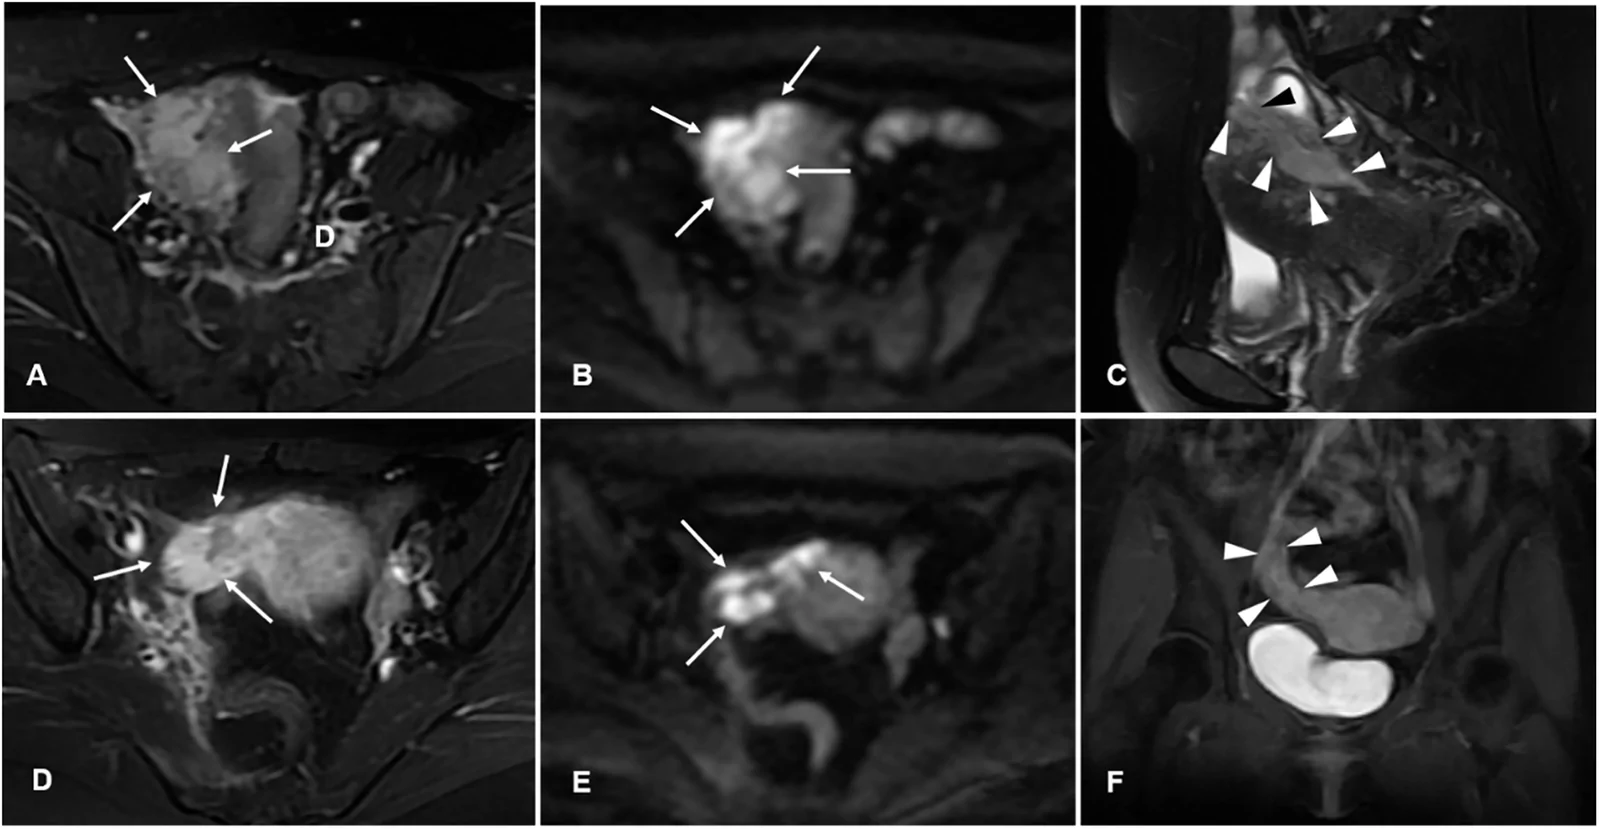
LG-ESS from pelvic MRI of a 43-year-old (A–C) woman and a 53-year-old (D–F) woman. Axial T2WI (A) and DWI (B) images showing ill-defined hyperintense lesions in the right posterior uterine wall and parametrium (arrows). Sagittal T2W image (C) revealing tumor extension beyond the uterus involving the right ovary and parametrial vessels (arrowheads). Axial T2W (D) and DW (E) images demonstrating ill-defined hyperintense lesions predominantly in the right parametrium with uterine wall involvement (arrows). Coronal T1-weighted contrast-enhanced image (F) displaying tumor extension along the parametrial veins
